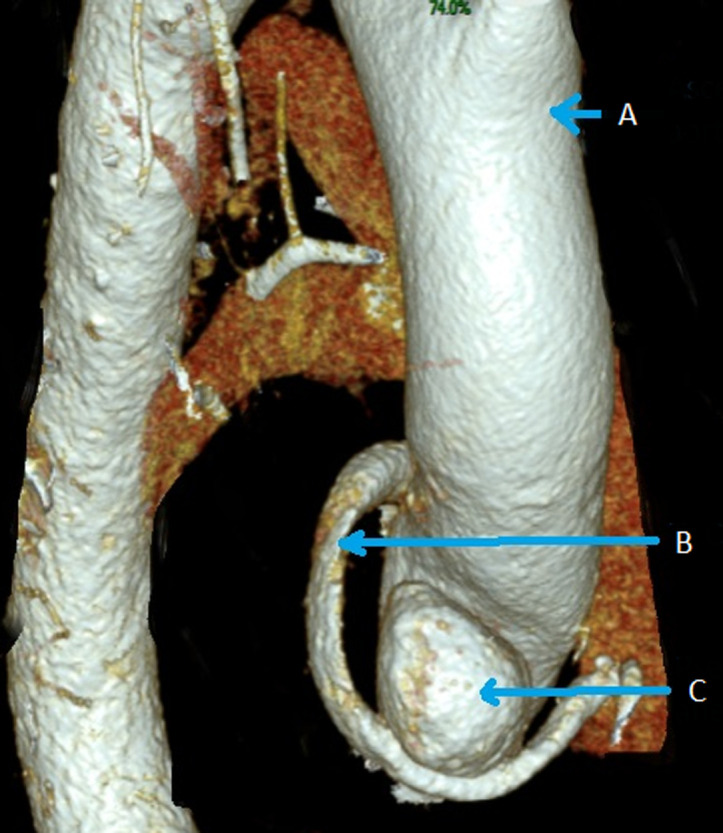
pre-operative computed tomography angiography scan showing rapidly increasing prostheto-prosthetic pseudoaneurysm: A) ascending aorta; B) T-Cabrol tube; C) pseudo aneurysm
